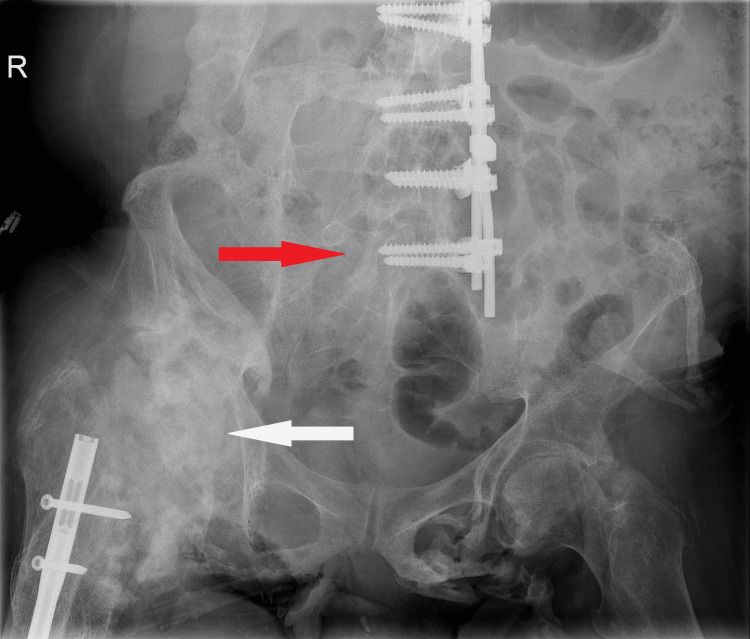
Pelvic radiograph showing the spinal instrumentation (red arrow), the femoral nail and the severe heterotopic ossifications, and the ankylosis of the right hip (white arrow).
